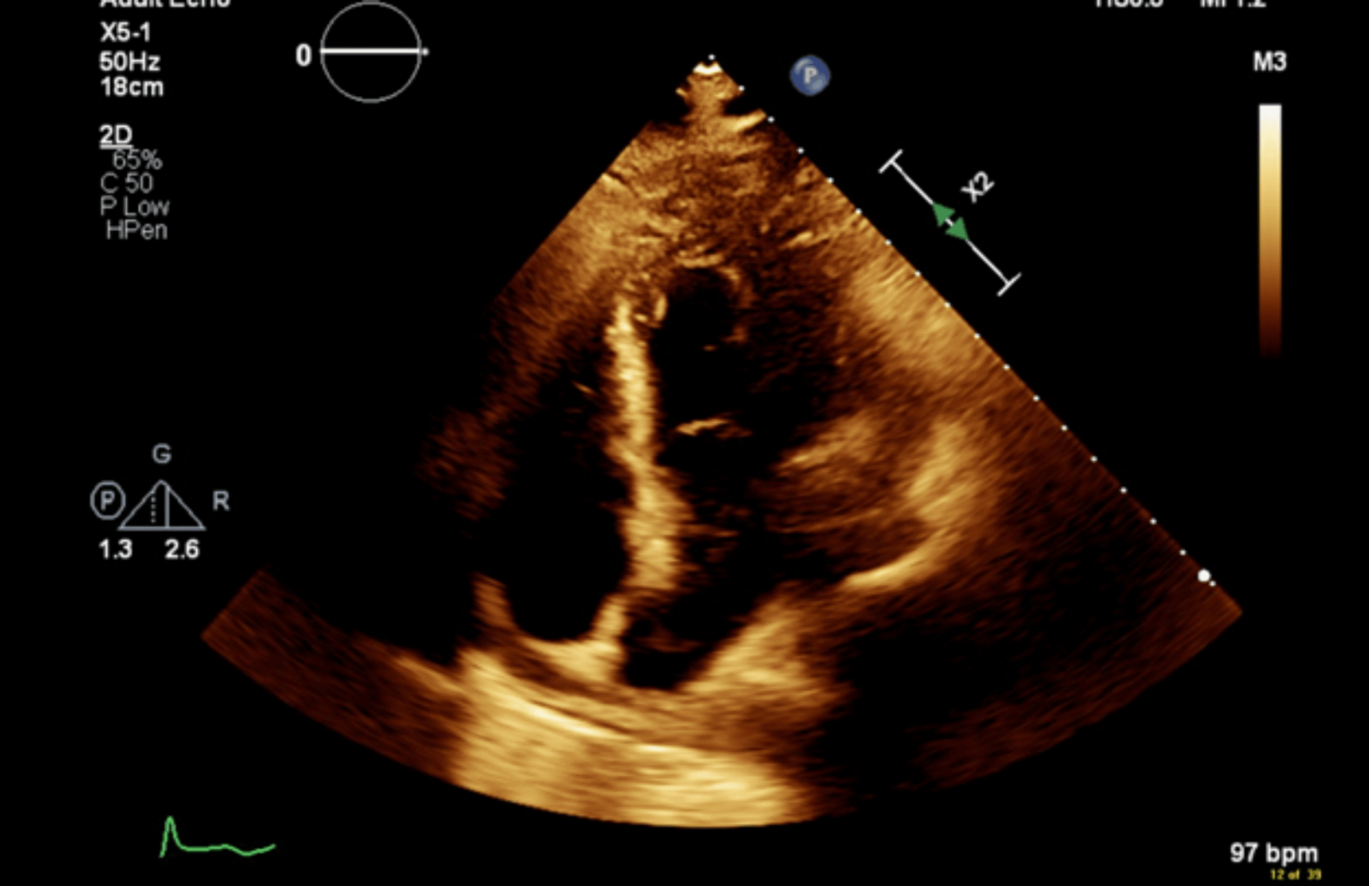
Transthoracic echocardiogram (TTE) performed on day 5 prior to initiation of intrapleural fibrinolytic therapy, showing no pericardial effusion. This study served as a baseline to assess cardiac involvement. The subsequent appearance of pericardial effusion on day 10 reinforces the importance of follow-up imaging in patients receiving intrapleural fibrinolytics near cardiac structures.
